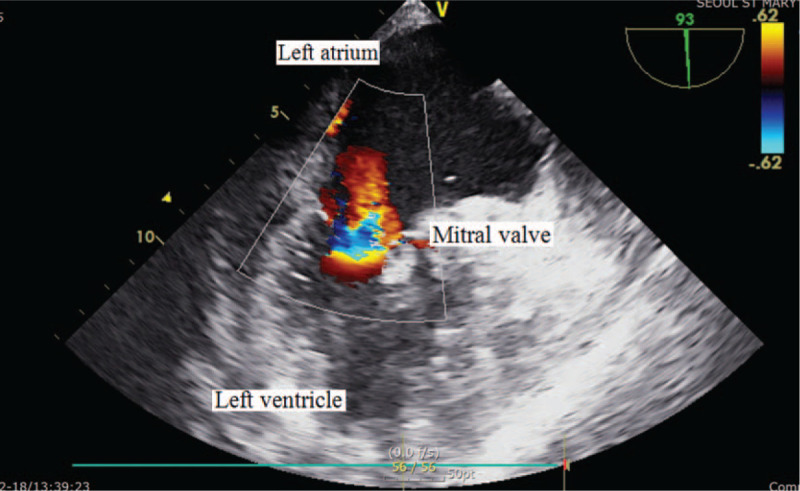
Severe mitral regurgitation seen on transesophageal echocardiography.
